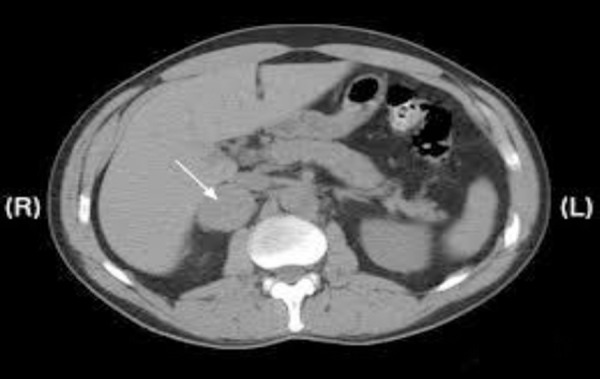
Adrenal tumor on abdominal CT*.
